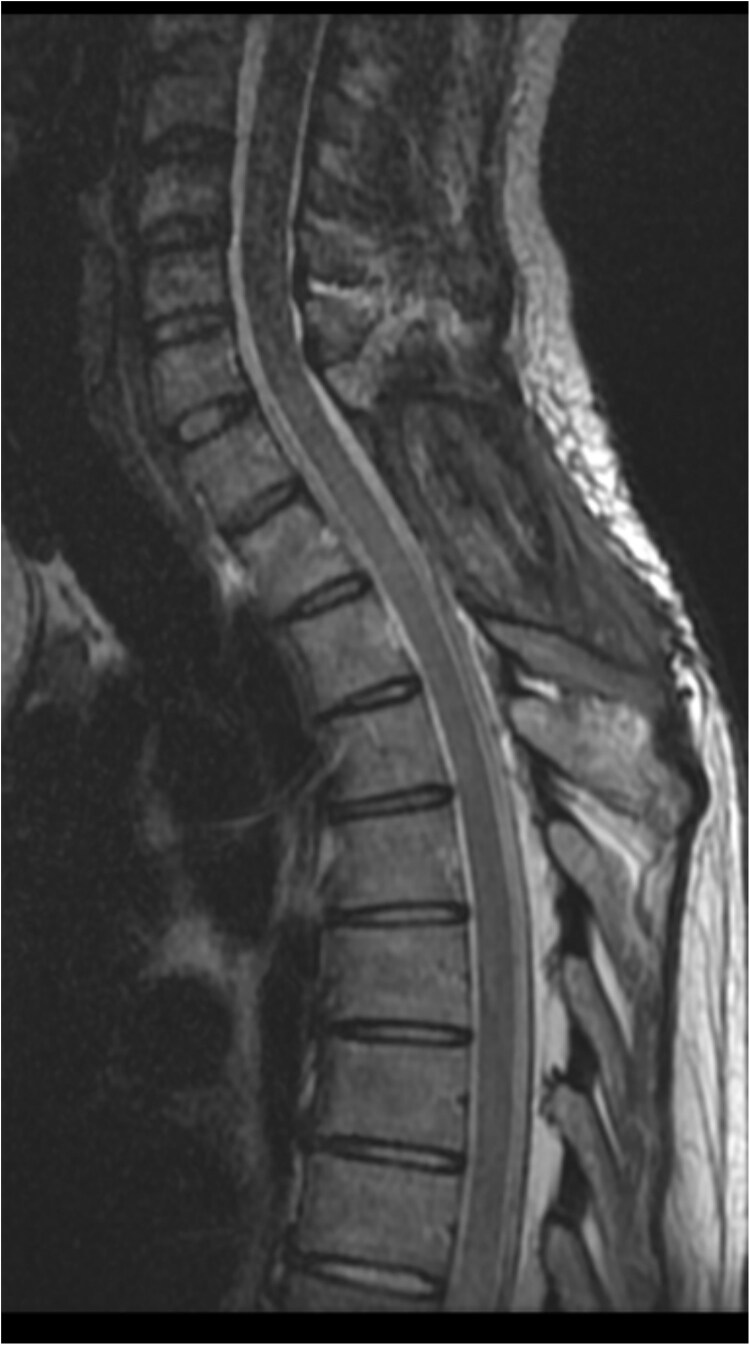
Eight months postoperative T2-weighted sagittal views MRI of the cervical spine.
